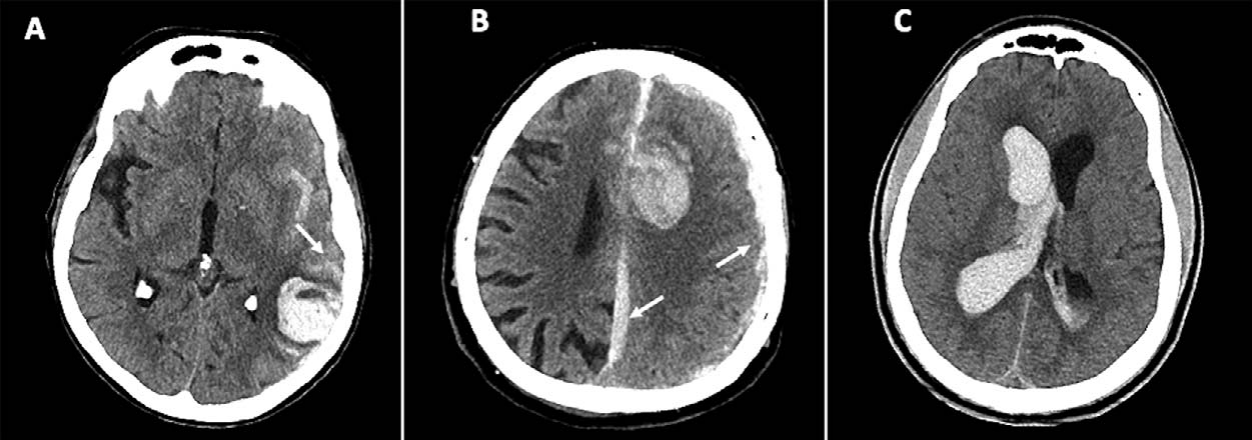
Examples of extra-parenchymal haemorrhagic NCCT findings suggestive of secondary causes of ICH. (A) Haemorrhage in the left temporal lobe with associated subarachnoid haemorrhage in the sulci and the Sylvian fissure (arrow), suggestive of arteriovenous malformation. (B) Haemorrhage in the left frontal lobe with associated subdural haemorrhages of the falx cerebri and the left hemispheric convexity (arrows), suggestive of arteriovenous malformation or cerebral venous thrombosis. (C) Isolated intraventricular haemorrhage suggestive of an arteriovenous malformation. Abbreviation: NCCT = non-contrast CT.
